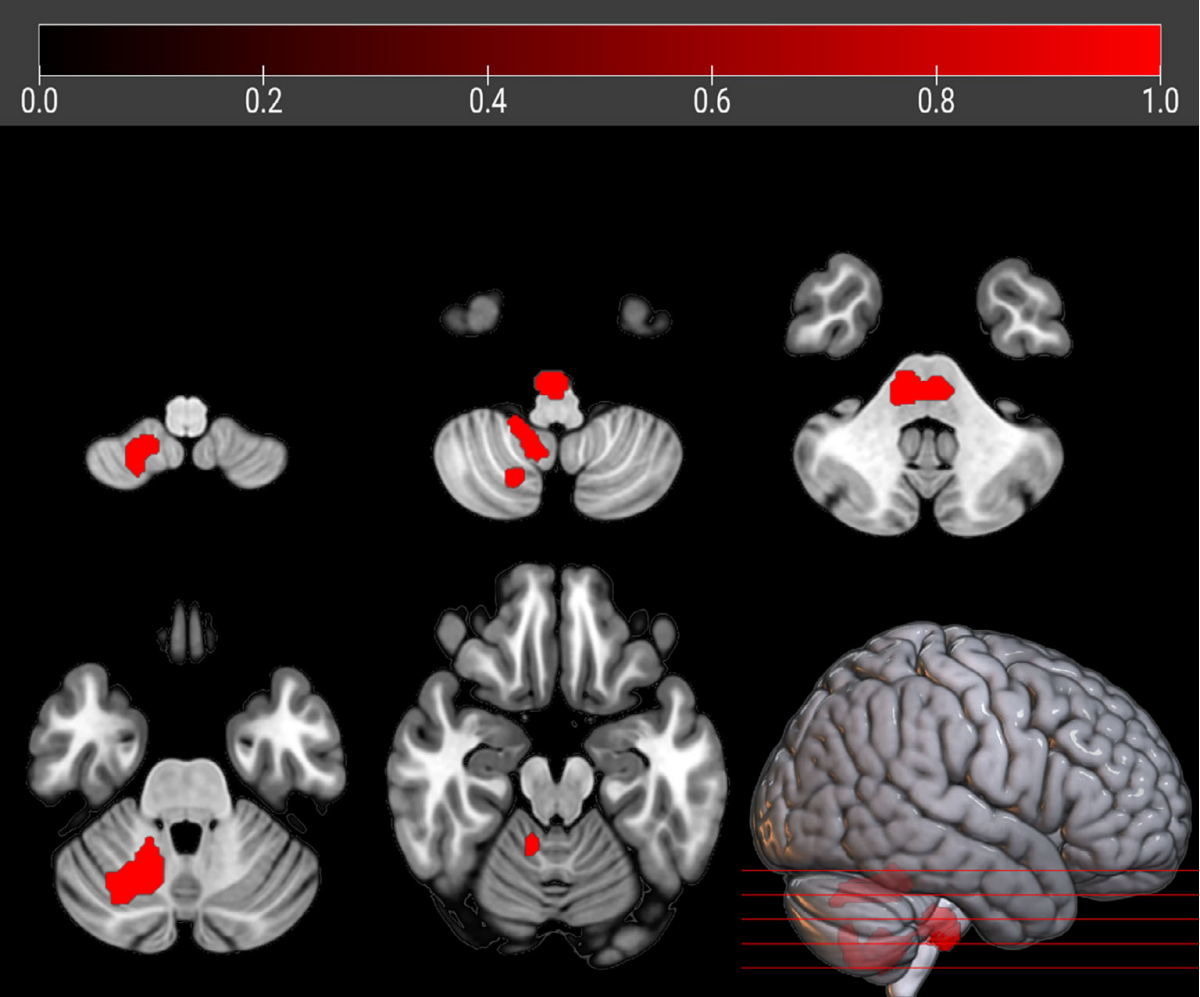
The regions showing a statistically significant relative hypermetabolism in *FUS*‐ALS patients as compared to HC after adjusting for age at PET and sex are marked in red and are reported on axial sections of a brain Magnetic Resonance Imaging template and on the brain surface of a glass brain rendering (bottom right).
